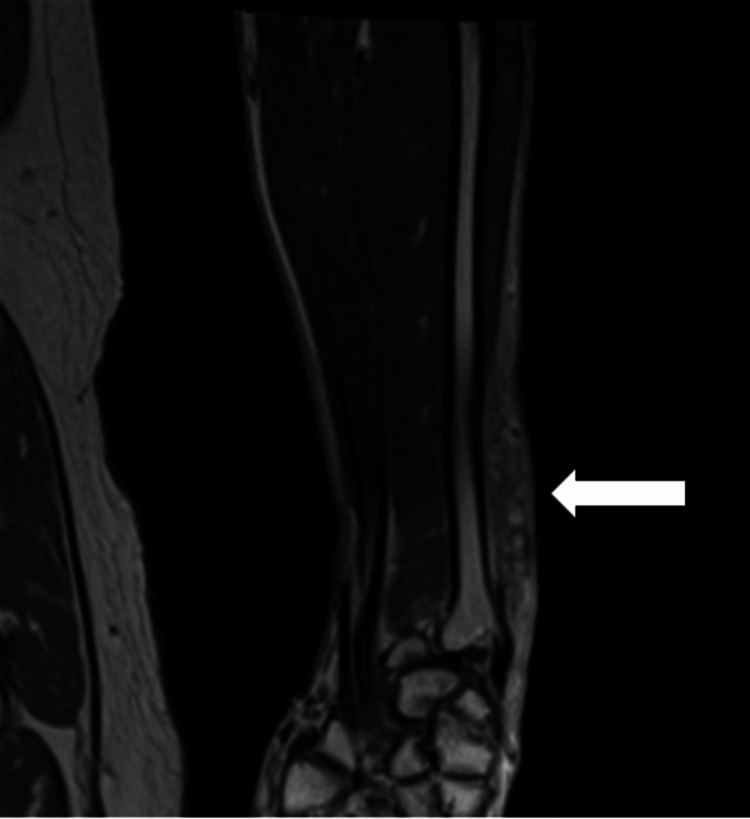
MRI showing inflammatory changes and edema in the soft tissues of the left forearm
